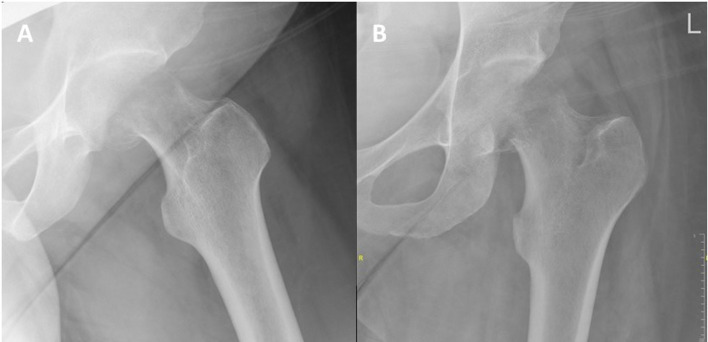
Lateral (A) and anteroposterior (B) radiographs of the left hip show a displaced sub-capital femoral neck fracture.
